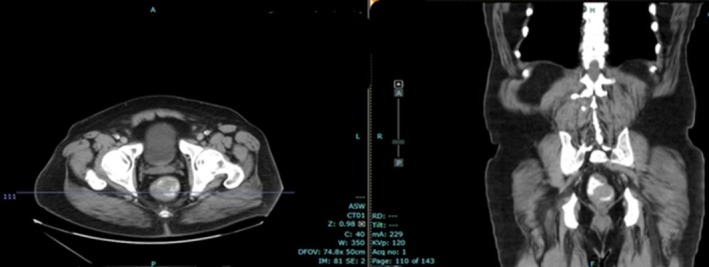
CT scan image of recurrence.
